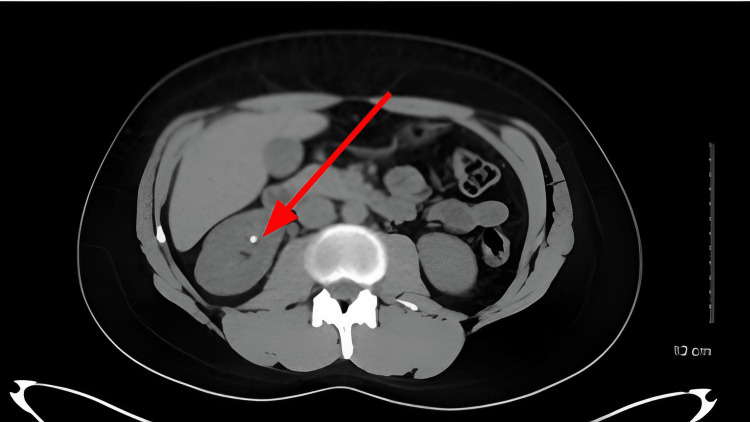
CT image with left renal stone.
